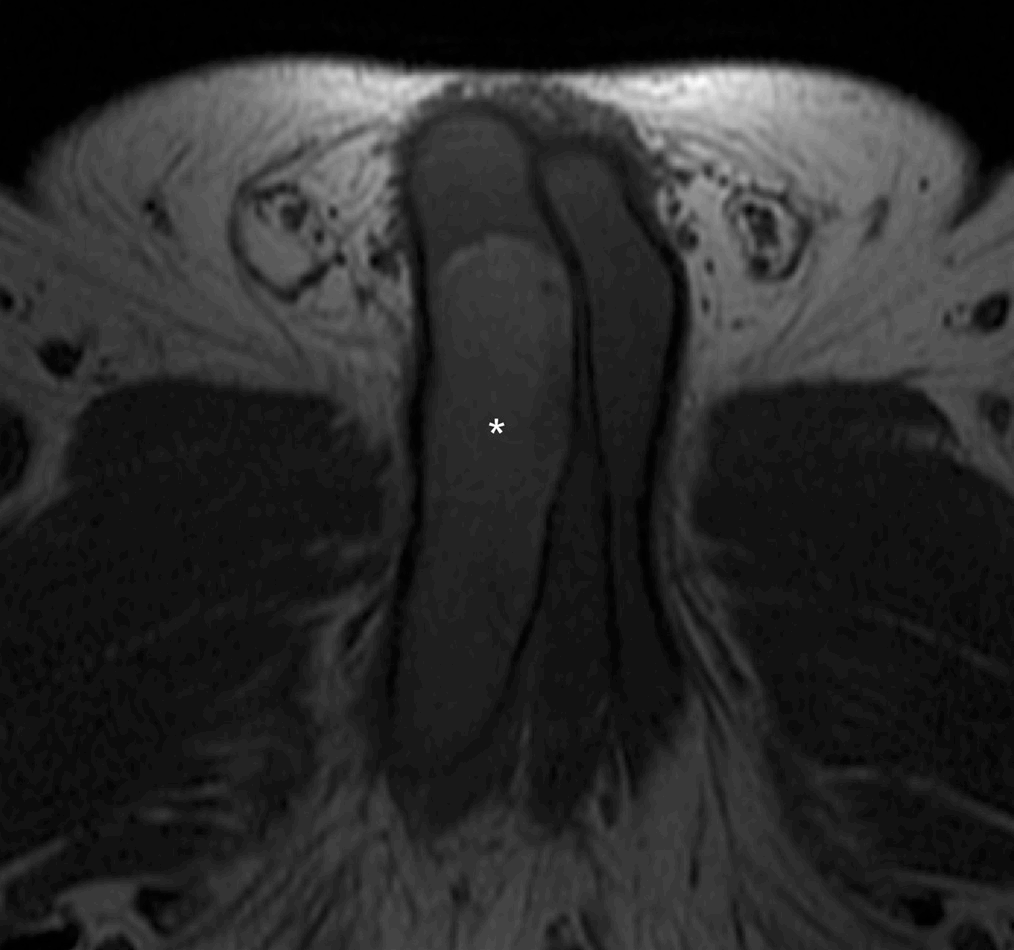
MRI (1.5 T) at the base of the penis. Axial view *T*_1_ weighted image shows the enlarged right corpus cavernosum, which is T1 hyperintense (*) comparing to the surrounding corpora. The left corpus cavernosum is displaced and compressed.
